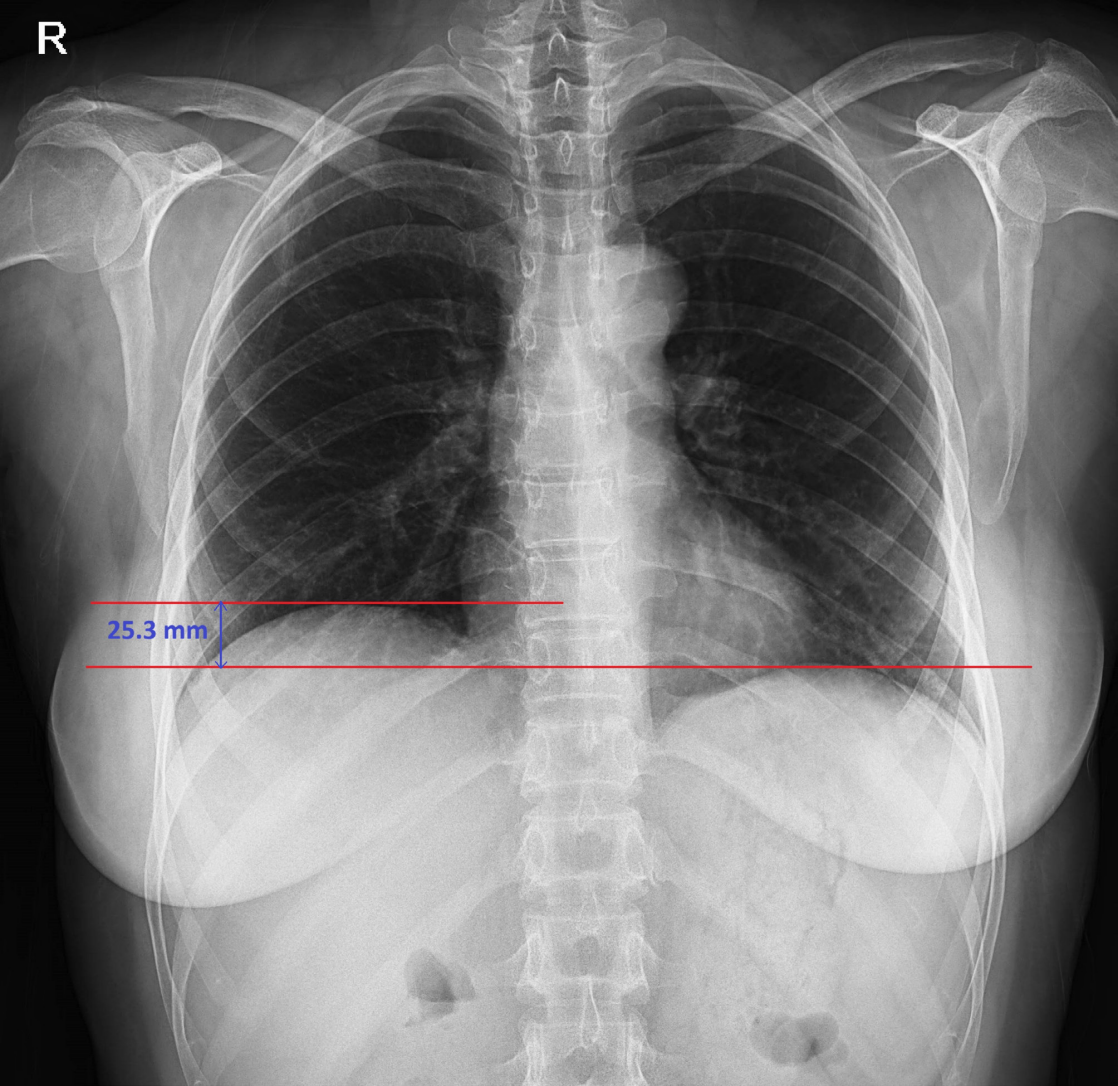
Chest radiograph demonstrating elevated right hemidiaphragm (25.3 mm measurement shown with calliper).
